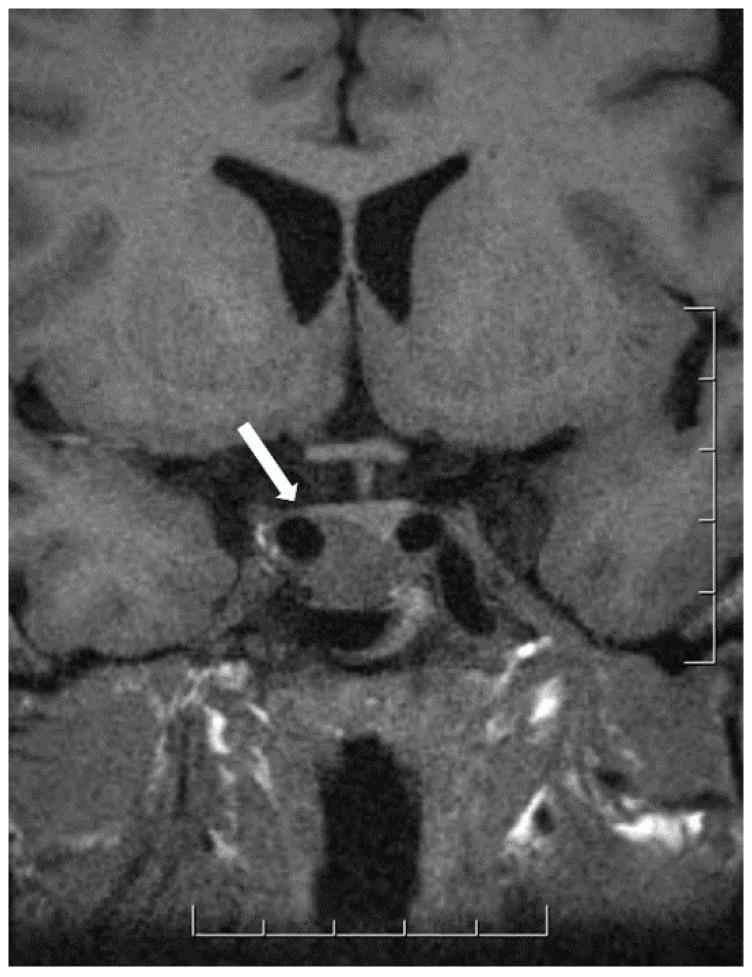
Magnetic resonance imaging of mammosomatotroph tumor. The sella turcica is enlarged and contains a tumor that compresses the normal gland upwards and to the left; the tumor infiltrates laterally to invade the right cavernous sinus (white arrow).
